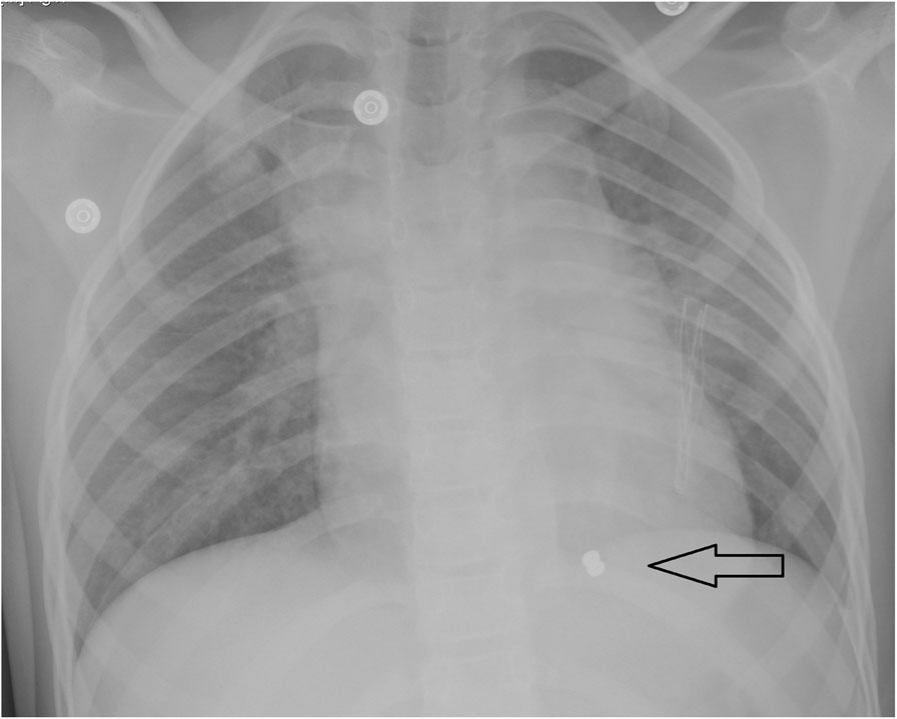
Postero-anterior chest X-ray showing a radio-opaque shadow (arrow) at the apical part of the heart more toward the right ventricle
